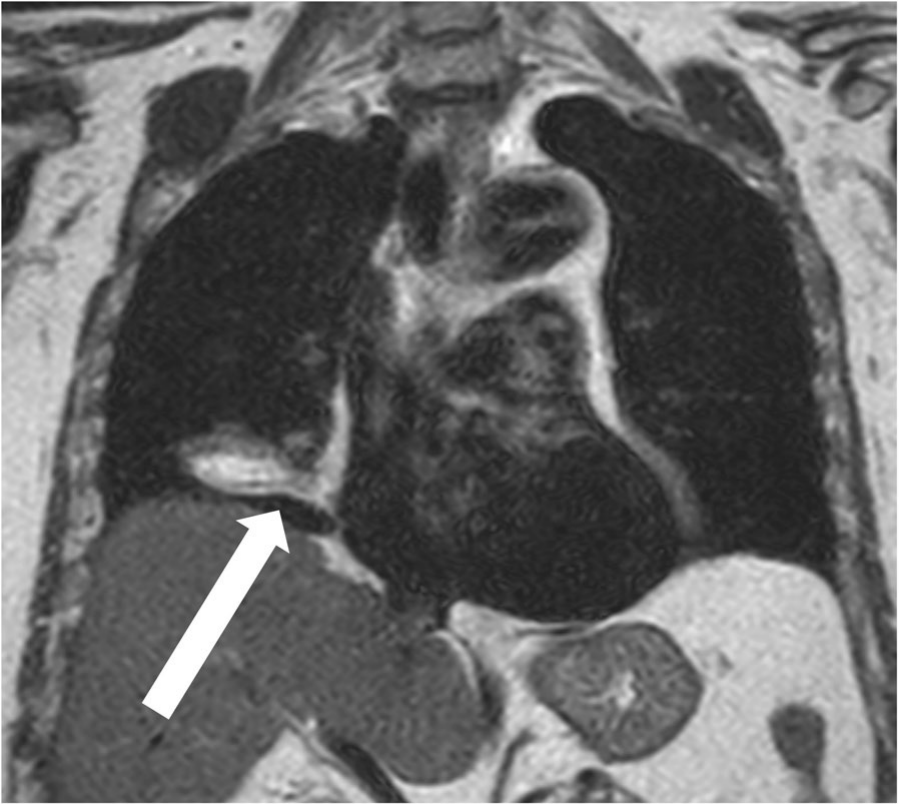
Chest magnetic resonance imaging (MRI) findings. A mass with mixed fat and soft tissue density can be observed in the major fissure area between the middle and lower lobes of the right lung. The lesion appears to be contiguous with pericardial fatty tissue
